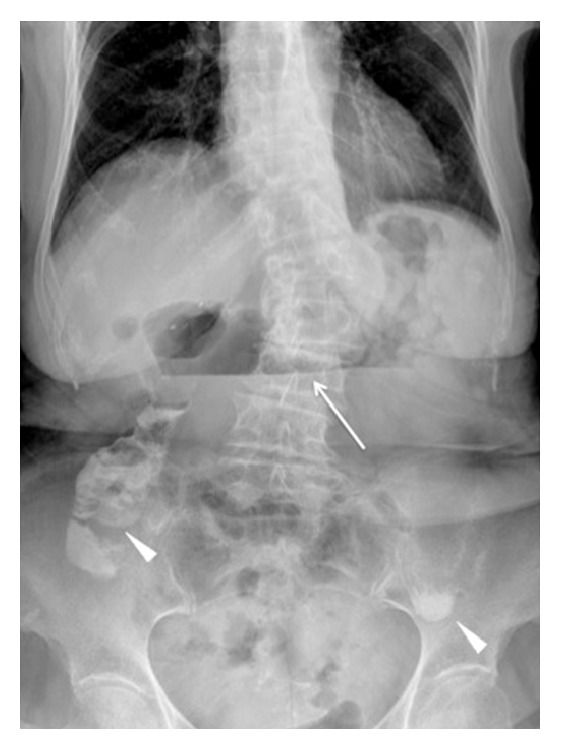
Plain abdominal radiography, performed at the time of emergency admission, shows the presence of a gastroduodenal air-fluid level (white arrow). The partial opacification of residual intestinal lumen (arrowheads) is due to assumption of oral contrast agent in a previous radiographic study performed one week before in another institution.
